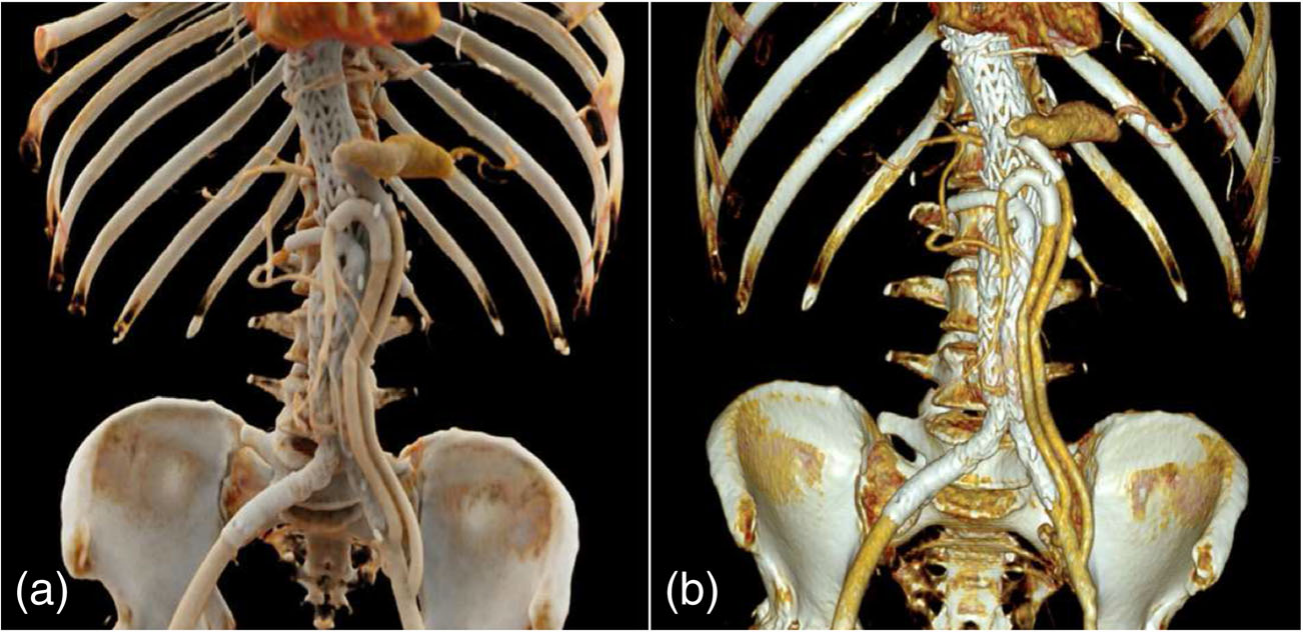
Contrast-enhanced 3-D CT images after endovascular stent graft treatment of the abdominal aorta with visceral debranching in a patient with infrarenal aortic and splenic artery aneurysm, shown with CR (a) and with VR (b)
